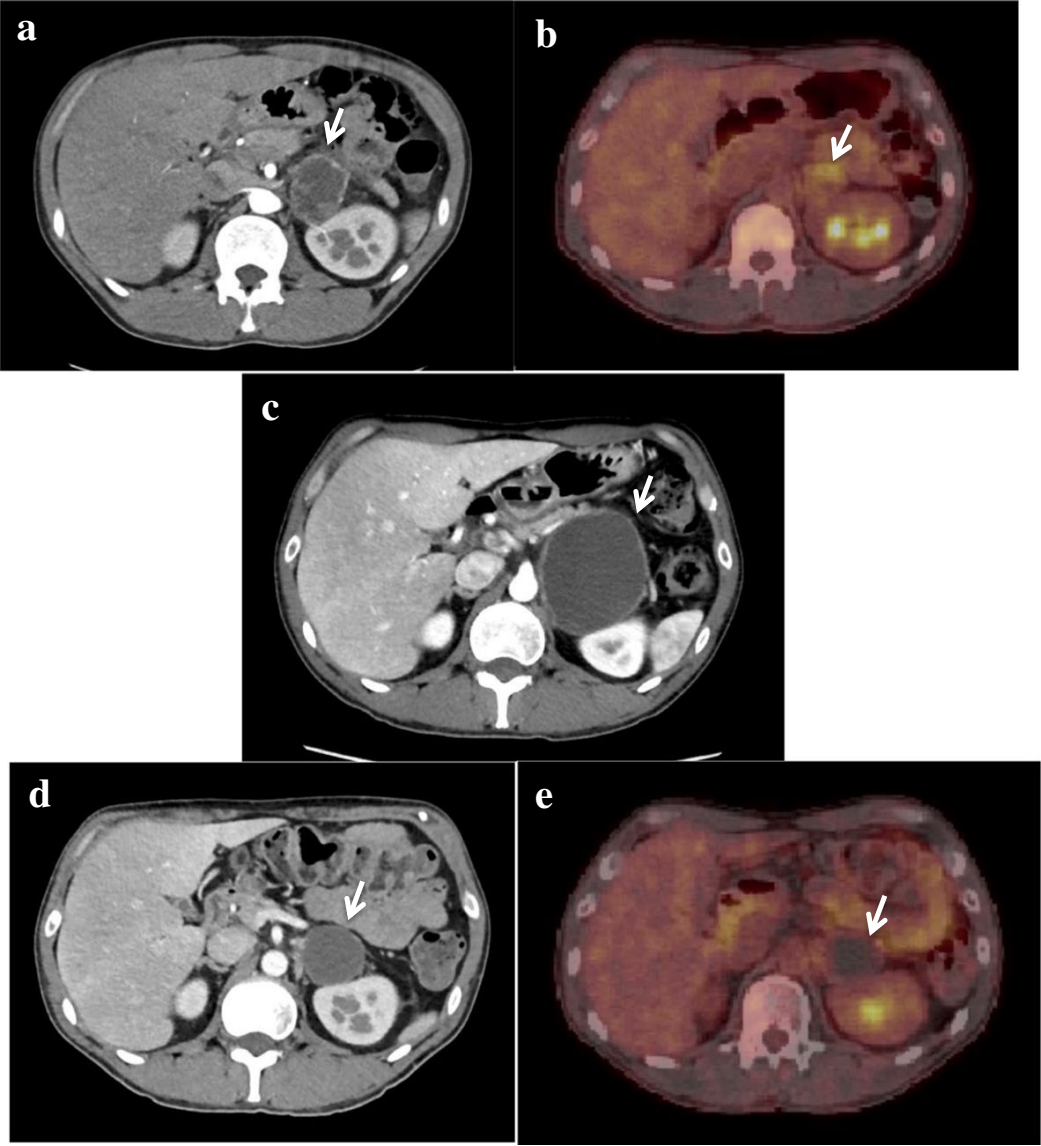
Distant metastasis to the left adrenal gland on imaging studies. a CT before the second radiotherapy with proton beam. b PET before the second radiotherapy with proton beam; remarkable fluorodeoxyglucose (FDG) uptake was found. c CT at the end of the second radiotherapy; larger but cystic and non-enhanced mass was observed. d, e CT and PET 8 months after the completion of the second radiotherapy; the size of the mass was remarkably reduced, and the blood flow nor the FDG uptake was not observed
